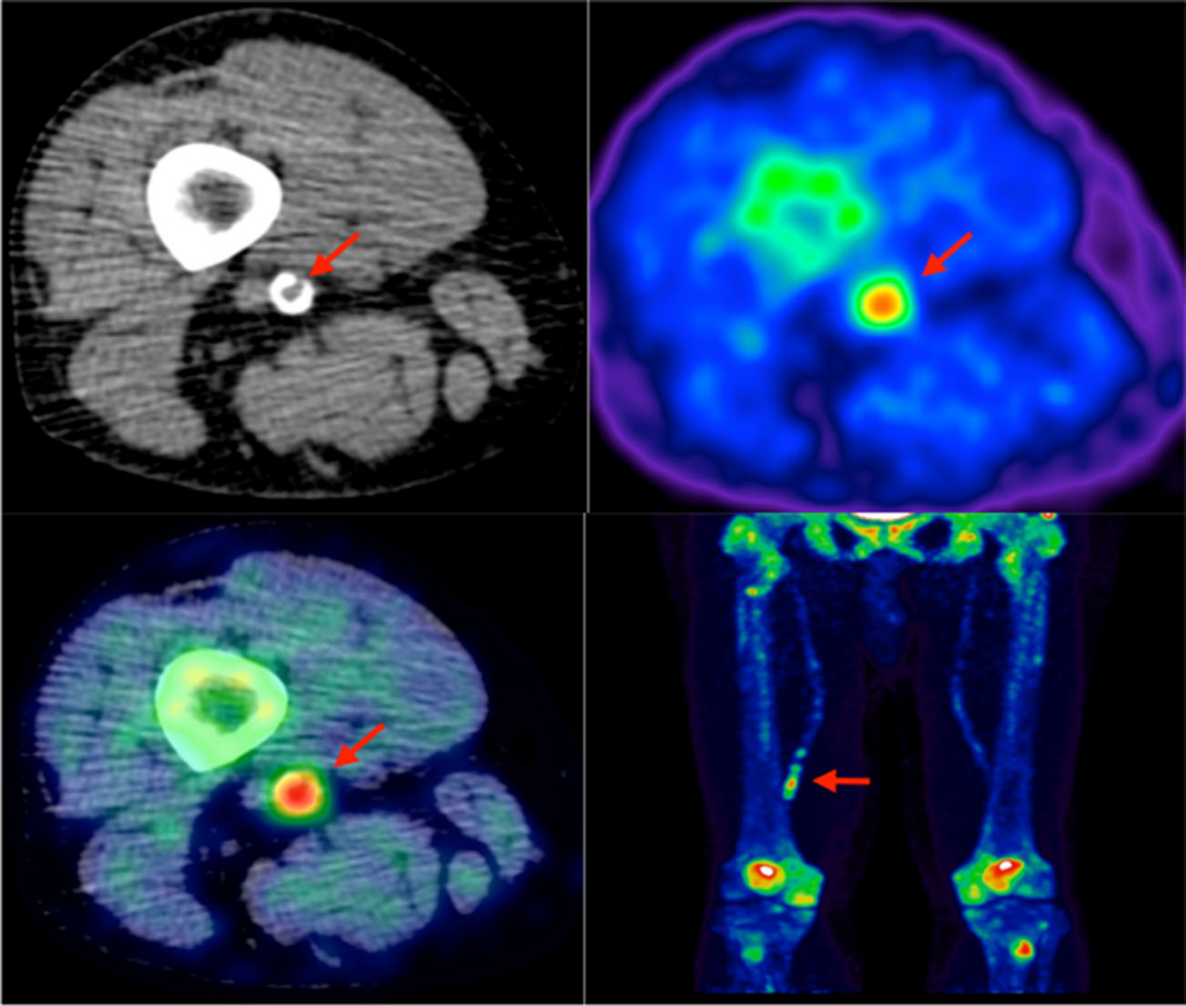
Lower limb ^18^F-NaF imaging: non-contrast CT (*top left*) with a rim of calcification of the vessel, ^18^F-NaF PET (*top right*), and fused ^18^F-NaF PET/CT (*bottom left*) of the superficial femoral artery (*arrow*) at the level of the adductor canal, demonstrating significant vessel uptake in this symptomatic patient. In addition, there is prominent uptake seen in the vessel at the same level on the coronal image (*bottom right*)
